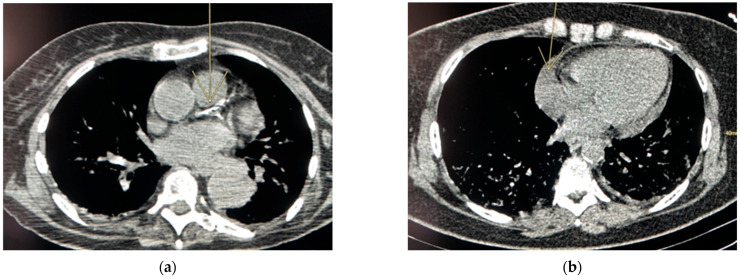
Calcified coronary atherosclerosis and pericardial effusion. (a) Chest CT of a 62-year-old woman performed in the context of suspected COVID-19 pneumonia with de novo discovery of calcifying atheromatosis. Coronary calcifications on left coronary artery ((left anterior descending artery and circumflex artery). (b) Chest CT of 58-year-old woman performed in the context of suspected COVID-19 pneumonia with novo discovery of a centimetric circumferential pericardial effusion.
